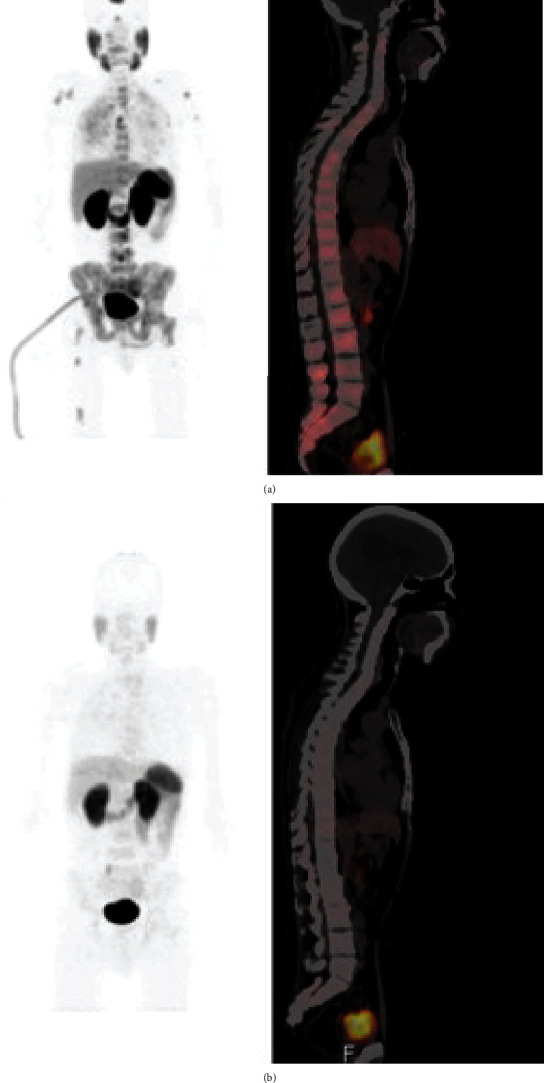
Response to ^225^Ac-PSMA-617 therapy in a representative prostate cancer brain metastasis. (a) Pretreatment ^68^Ga-PSMA PET/CT. (b) Restaging PSMA PET/CT scan after one cycle of ^225^Ac-PSMA. Reproduced with permission from Sathekge et al. [64].
